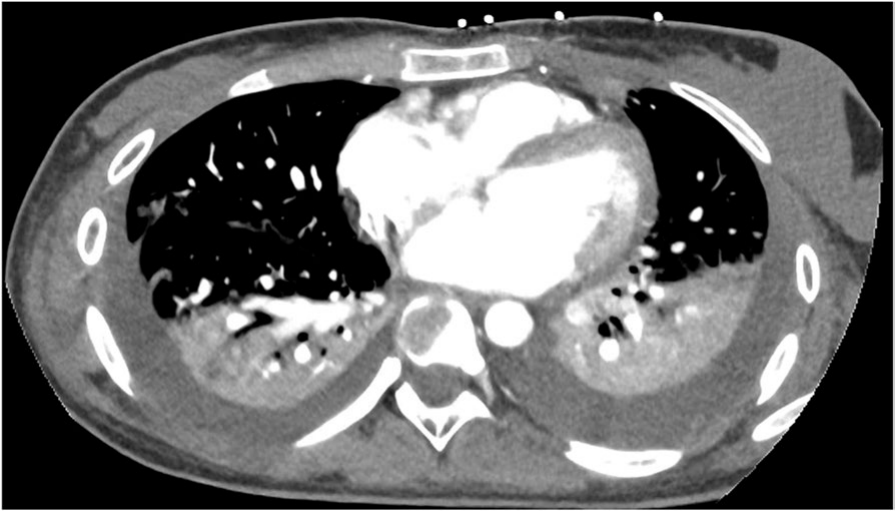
Toracic CT scan: hypodense nodular lesions, related to septic emboli. Moderate pleural effusion
